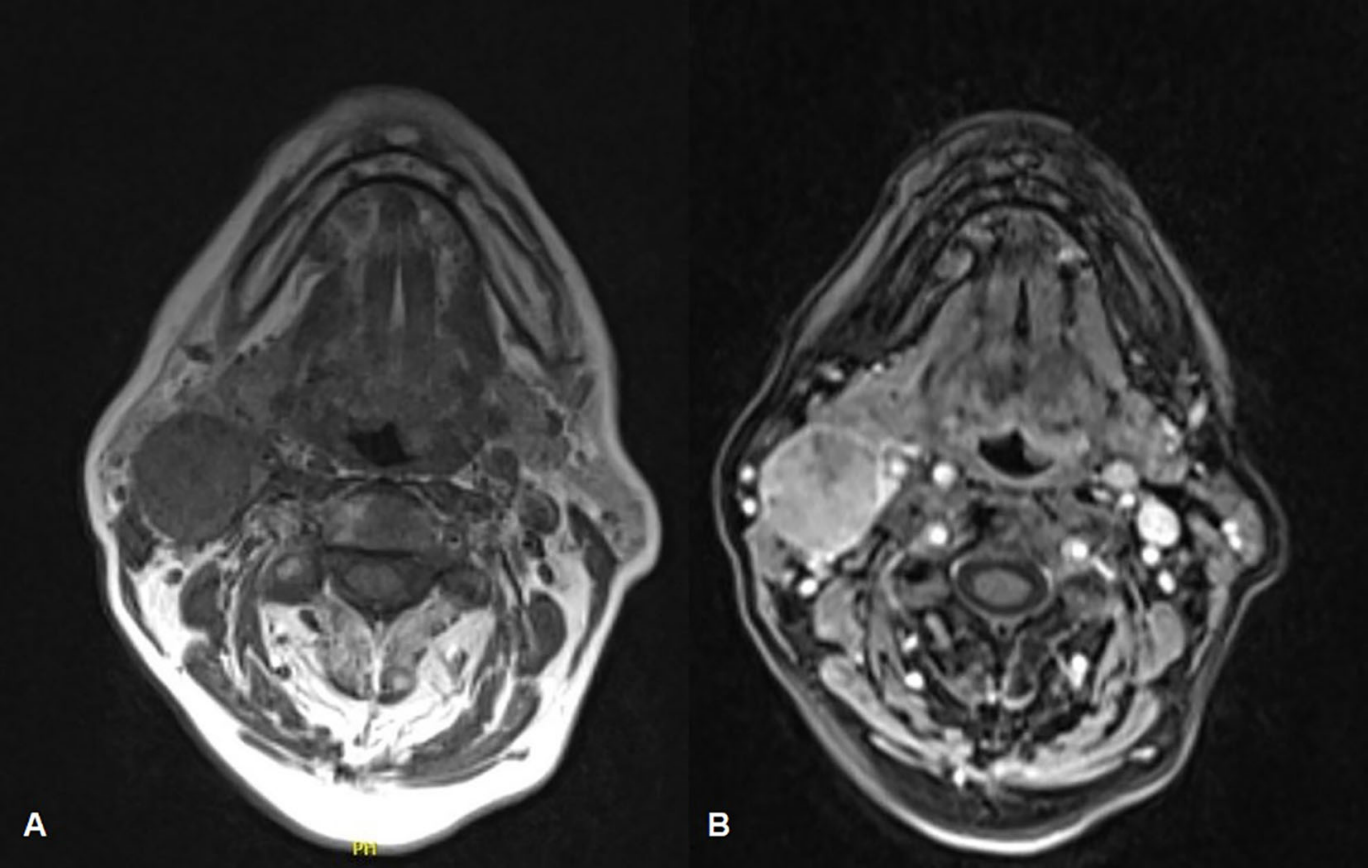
Face-bone MRI showing the primitive neoplasm at the right tonsillar pillar (1 cm), along with numerous lymphadenopathies located bilaterally in the lateral cervical region, within which necrotic-colliquative phenomena are evident (A). A modest post-contrast impregnation is evident at the right tonsillar pillar and the most prominent lymphadenopathy is noted at the right cervical area, to the level IIa (B).
